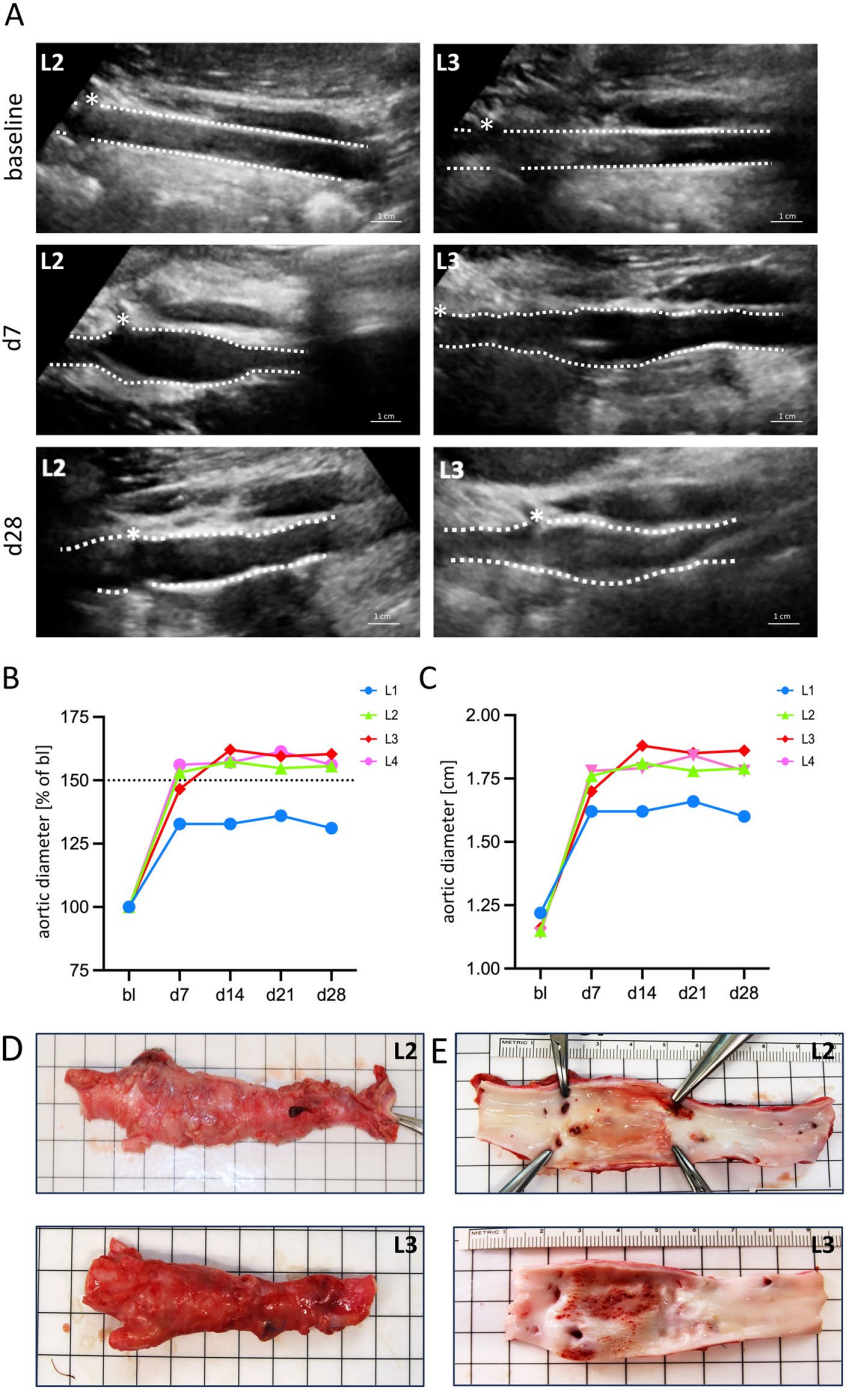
Aortic dilation after AAA surgery. (A) Representative sonographic images of the infrarenal aorta at baseline (bl), seven (d7), and 28 (d28) days post AAA surgery. Dashed lines indicate the abdominal aortic wall, *marks the right renal artery. (B) Aortic dilation in percent as normalized to baseline (bl) diameter. Baseline diameter was assessed 2 cm distal to the renal artery. (C) Respective aortic diameters in cm. Explanted abdominal aortas 28 days after AAA surgery (D) before and (E) after longitudinal dissection revealing subluminal bleeding and an increase in vessel wall thickness.
